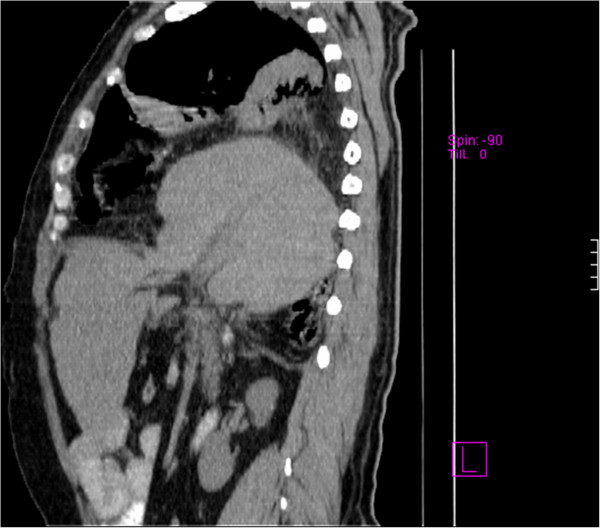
Computed tomography (CT) scan showing intrathoracal displacement of two-thirds of the liver.
